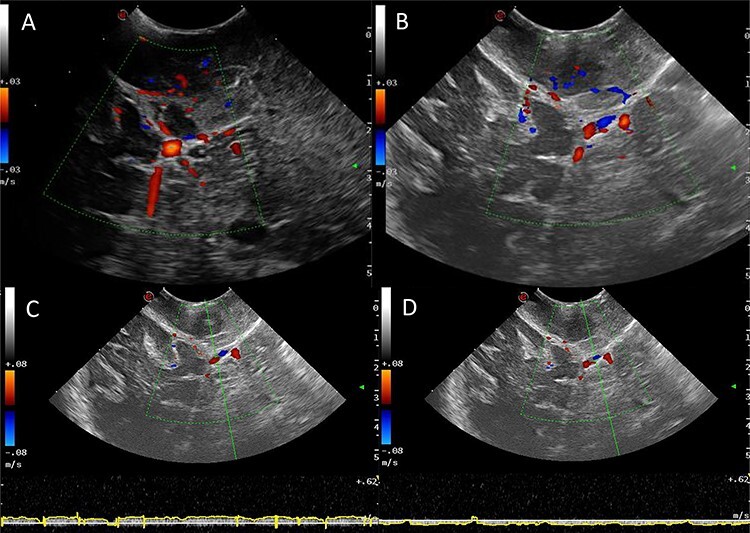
Intraoperative images at the end of resection; Duplex US images (2D image with overlay of colour Doppler) of the initial appearances of the straight sinus (A) and appearance at the end of resection (B); a bidirectional flow in the straight sinus is apparent after tumour resection, this was not present prior to tumour debulking; the Triplex Doppler confirms the bidirectional flow in the straight sinus (C and D).
